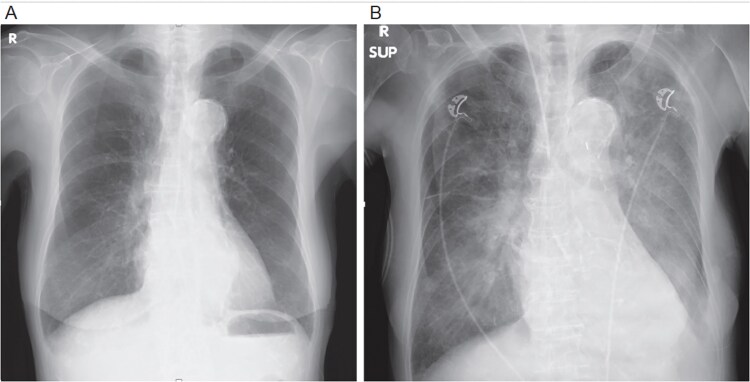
Chest X-ray: (A) immediately and (B) at the time of decompensation.
